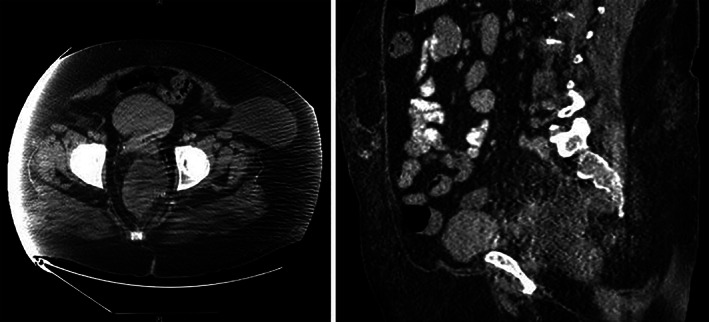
CT chest/abdomen/pelvis: Anorectal mass axial and sagittal view
